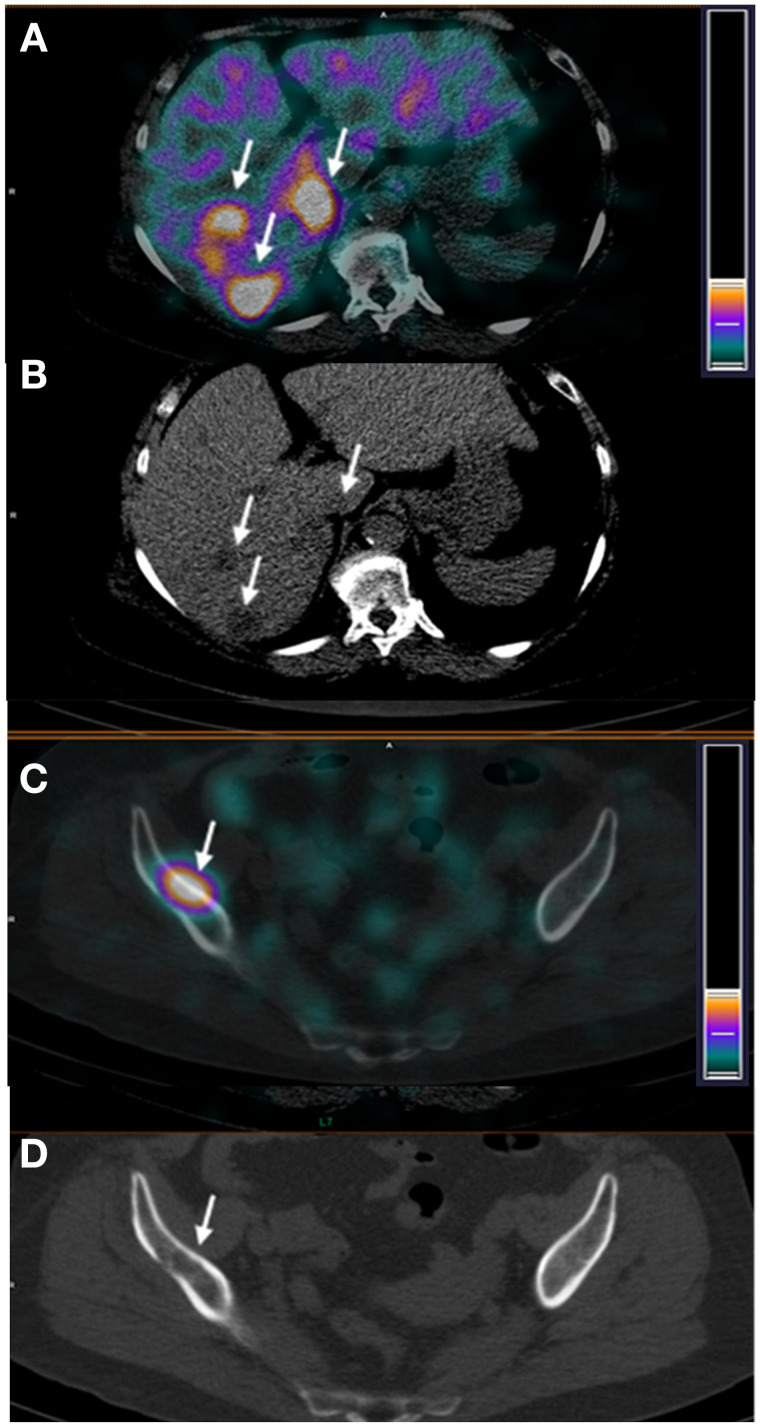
Patient 27. SPECT/CT (A) and CT (B) images of hepatic lesions. SPECT/CT (C) and CT (D) images of iliac bone lesions. Arrows point at lesions.
